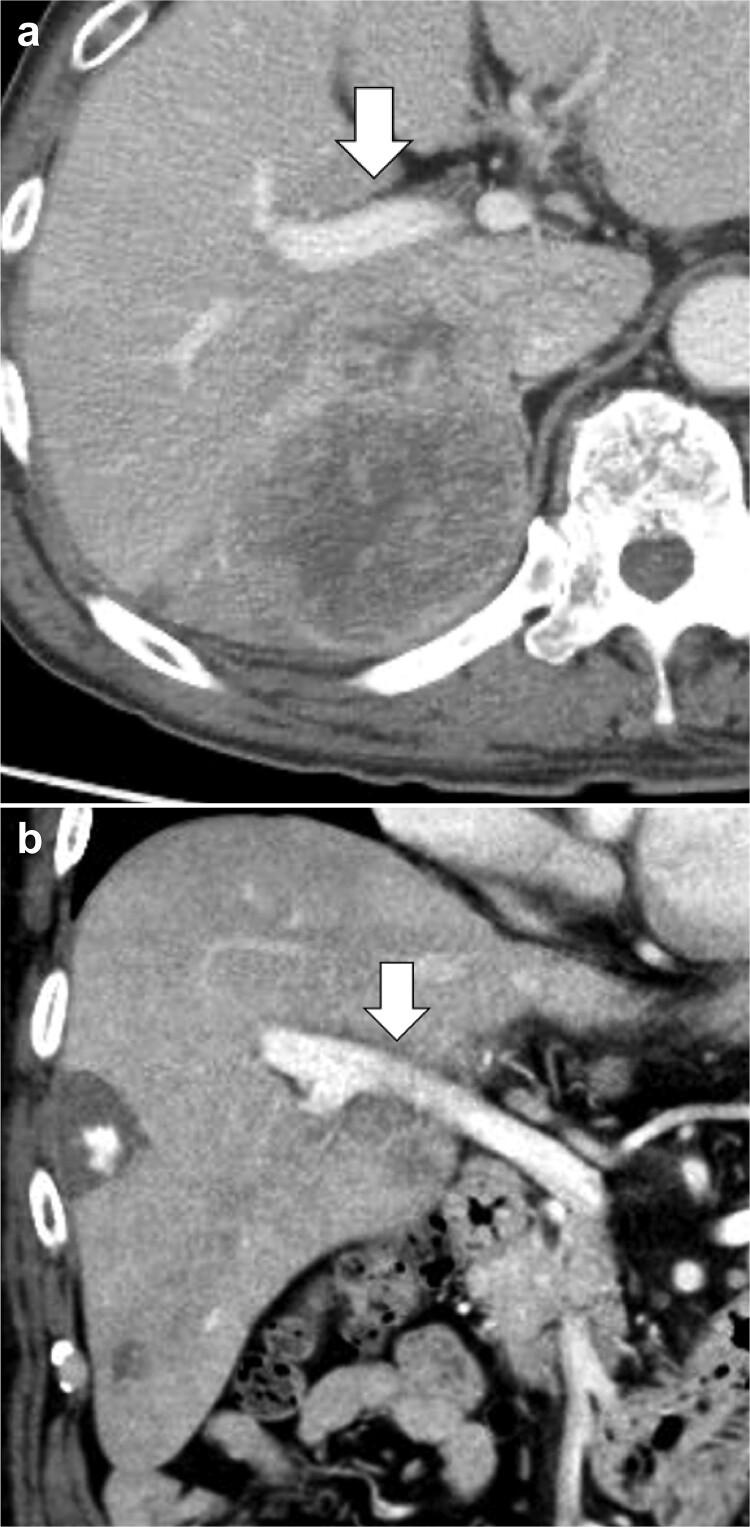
Initial abdominal contrast-enhanced CT scan. (a) A 9.1 × 9 cm large multinodular fused tumor was seen in the posterior region, predominantly in the caudal paravalvular region. HCC was diagnosed based on the contrast patterns. (b) The tumor was indistinctly bound to the first branch of the portal vein.
